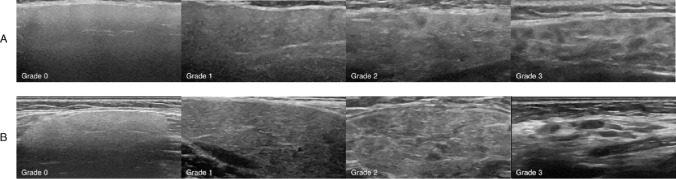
SGUS atlas. Ultrasound images examples of the four-grade semi-quantitative scoring system developed and validated by the OMERACT ultrasound working group: parotid gland (A) and submandibular gland (B) grade 0, normal parenchyma; grade 1, minimal change: mild inhomogeneity without anechoic/hypoechoic areas; grade 2, moderate change: moderate inhomogeneity with focal anechoic/hypoechoic areas; grade 3, severe change: diffuse inhomogeneity with anechoic/hypoechoic areas occupying the entire gland surface
